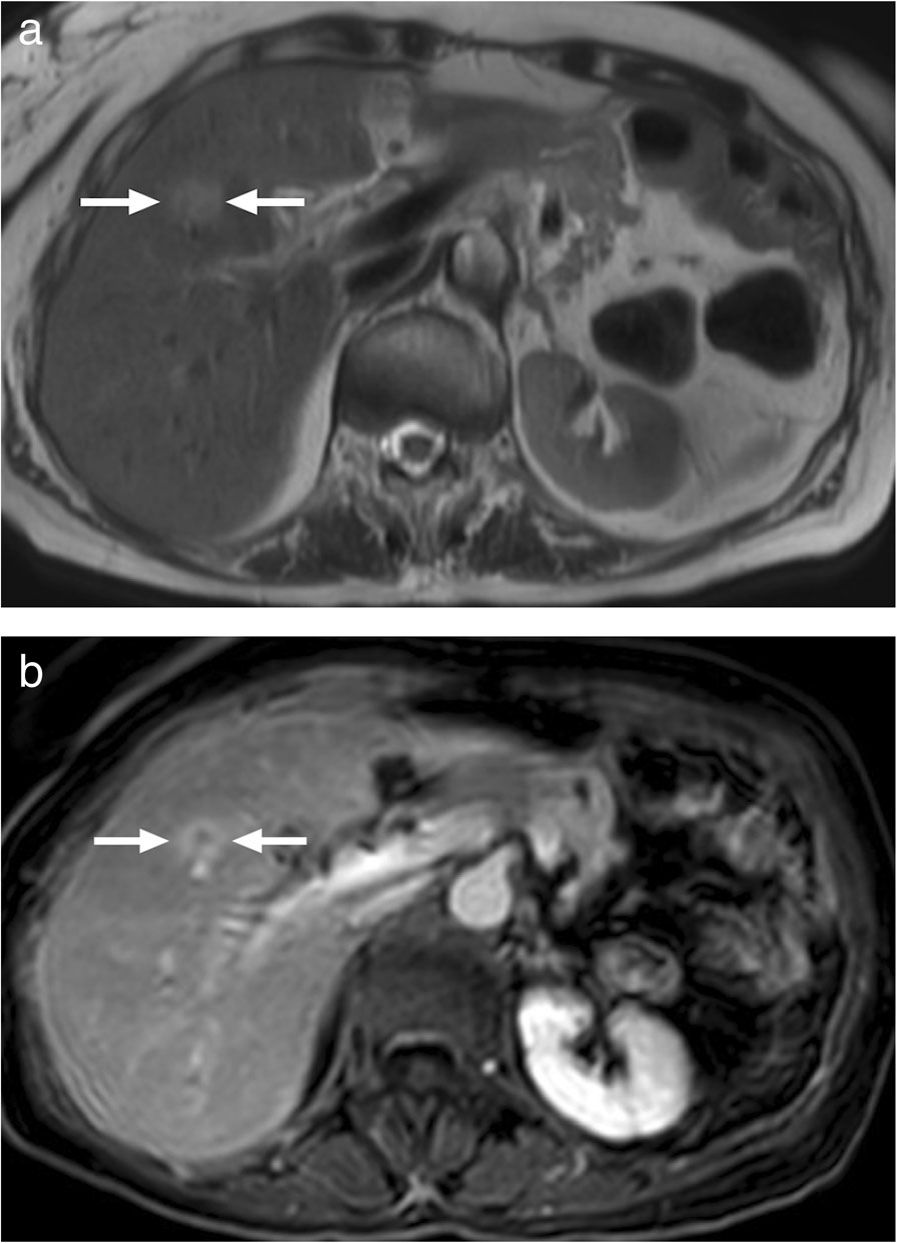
A 64-year-old female with previously surgically treated pancreatic adenocarcinoma now presenting with a hypoechoic focal liver lesion detected in outside US. Clinically the patient was in complete remission for her tumor and her prior abdominal scan was normal. She was asymptomatic and had no fever. a Axial plane T2W MR image demonstrates a moderately hyperintense focal lesion (arrows) in the right liver lobe. b Axial plane postcontrast T1W MR image at the late arterial phase demonstrates marked ring enhancement in the periphery of the lesion (arrows) which was considered to be highly suspicious for an adenocarcinoma metastasis. Percutaneous biopsy did not demonstrate any neoplastic cells but was interpreted as an abscess. Follow up CT scan (not shown) after intense IV antibiotic treatment showed complete disappearance of the lesion
